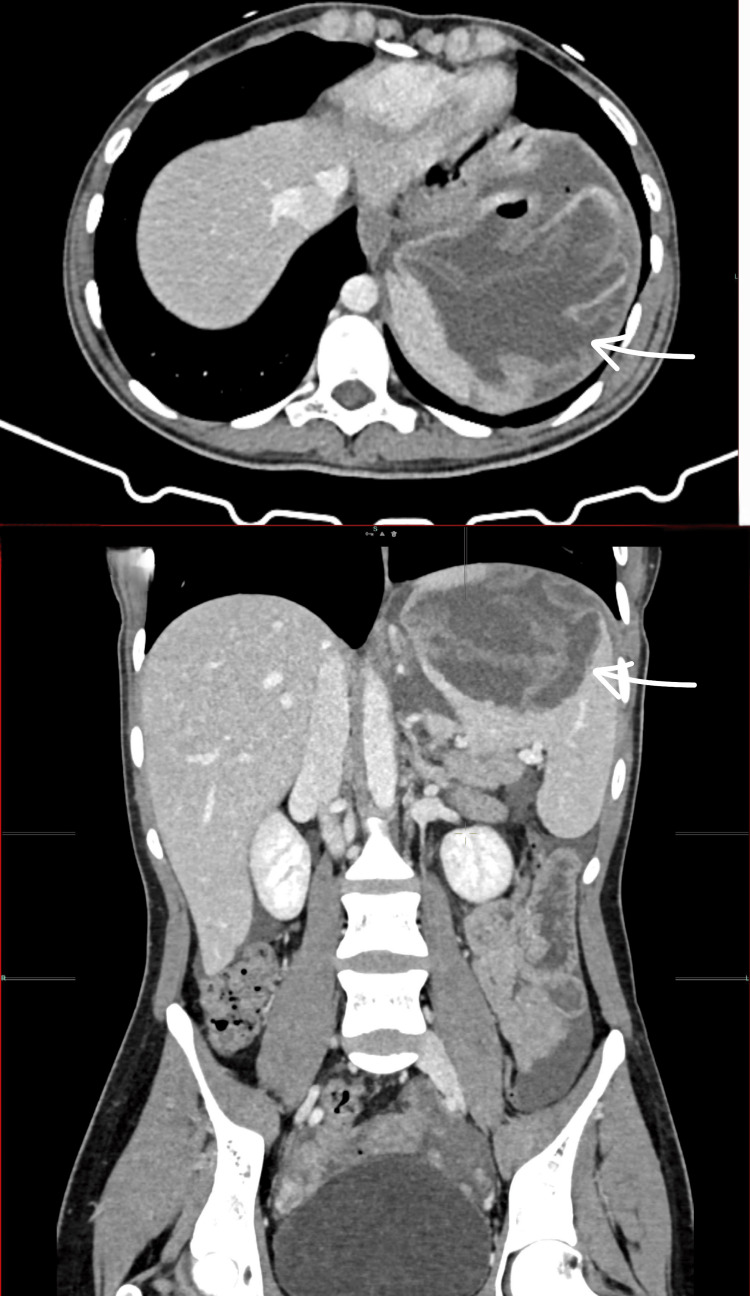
Two CT scout films of the patient at presentation showing a large complicated splenic cyst (18 × 15 × 12 cm) CT: computed tomography
